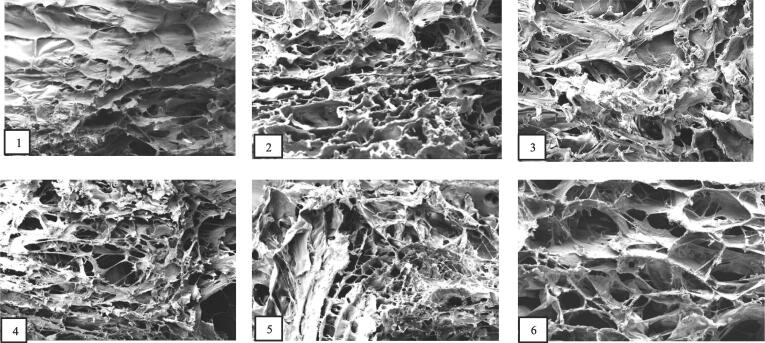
SEM images of acid soluble collagen extracted with the aid of ultrasound at different treatment time (0 min: 1, 5 min: 2, 10 min: 3, 15 min: 4, 20 min: 5 and 25 min: 6) from yellowfin tuna skin at 2000x magnification.
